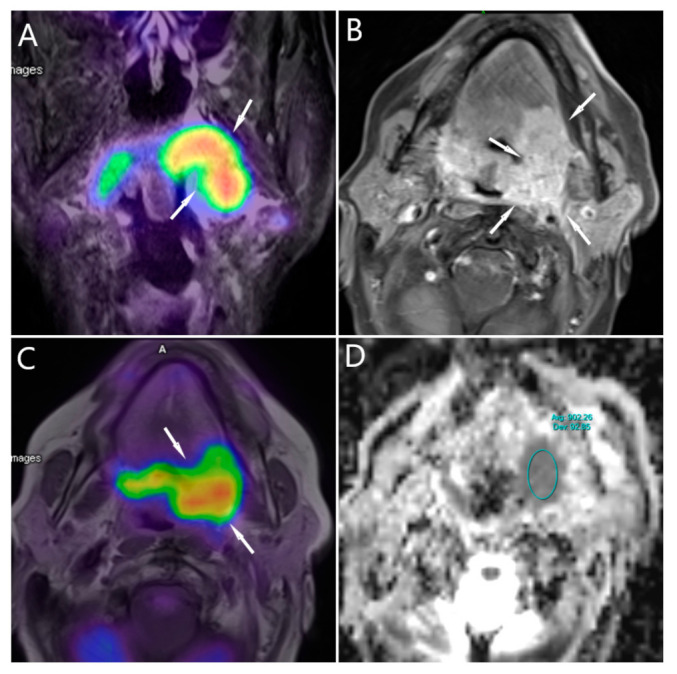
(A–D) and ^18^F-FDG measurements of 67 male patients with Oropharyngeal carcinoma. (A) T2-PET_tirm coronal MRI shows the intensive FDG accumulation (arrow). (B) T1-TSE-sagittal shows the extent of the tumor, lateral pharyngeal wall into the tongue root to the left tongue body (arrows). (C) T1-PET fused image shows the ROI within the tumor (arrows), and (D) DWI/ADC map showing the average and standard deviation of the ADC value.
